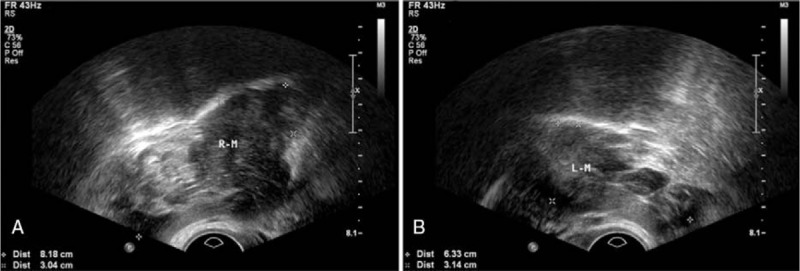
Preconceptional transvaginal ultrasonography (A) detected a heterogeneous and hypoechoic mass behind the right adnex (approximately 8.1 × 3.0 in size) and (B) left adnex (approximately 6.3 × 3.1 cm in size).
